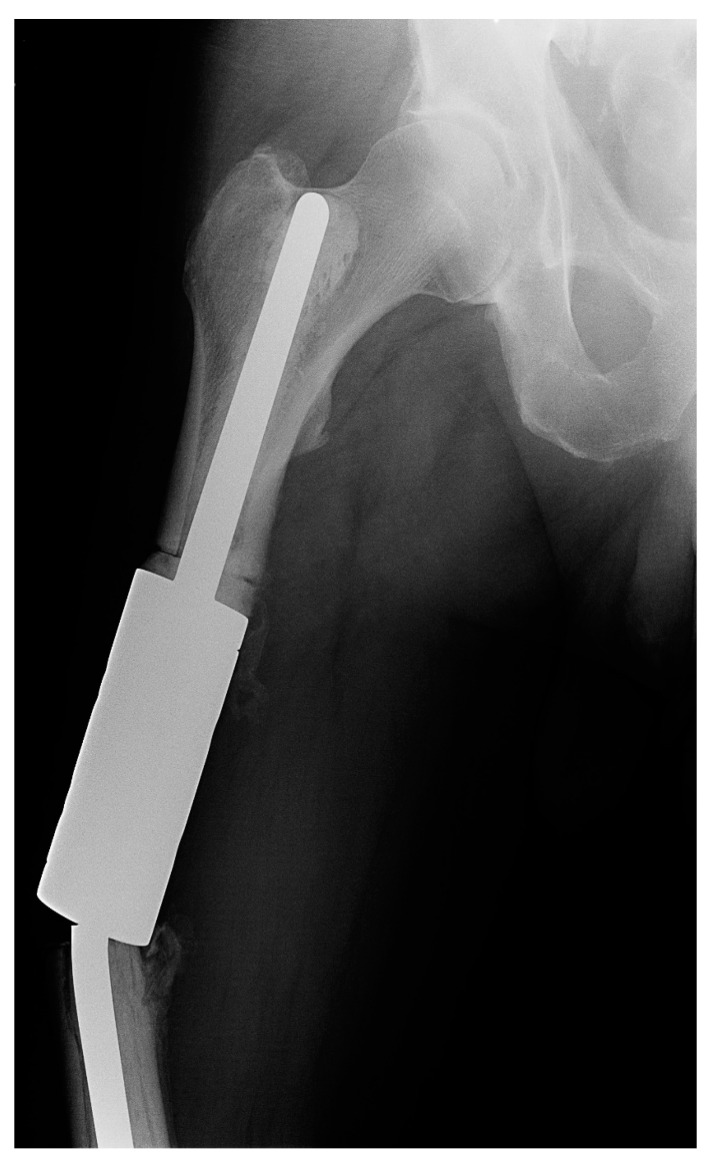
X-ray image of a fracture at the clamp-rod interface (type III failure) of an individual femoral diaphyseal implant for renal cell carcinoma oligometastatic disease. Revision intercalary endoprosthesis was performed. This image is from patient 8 in Table 1.
